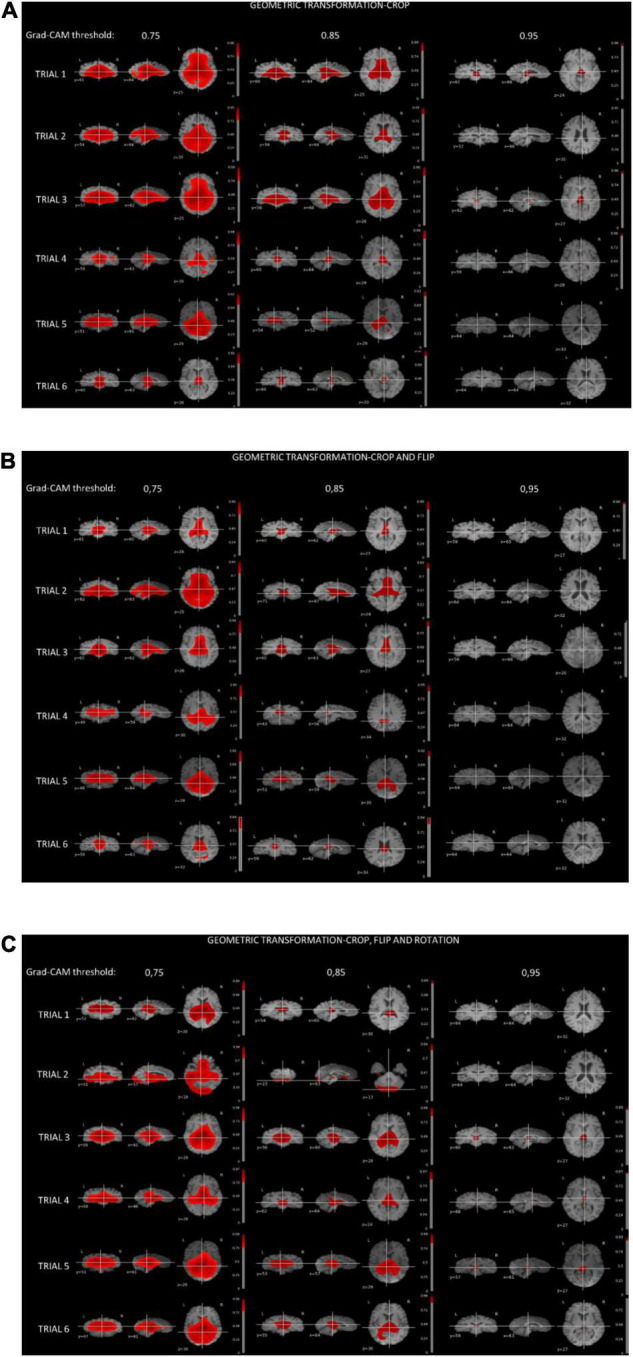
(A) Grad-CAM results of NPSLE patients in the six trials augmented with “crop” (A), “crop and flip” (B), and “crop, flip and rotation” (C). Each row displays thresholded mean grad-CAM maps obtained from the NPSLE test set of one of the trials (1 through 6) overlaid on a T1-weighted dataset. Each column displays a different Grad-CAM threshold: 0.75 (left), 0.85 (middle), and 0.95 (right). Within each column, data are shown in three different orientations: coronal (left), sagittal (middle), and axial (right).
